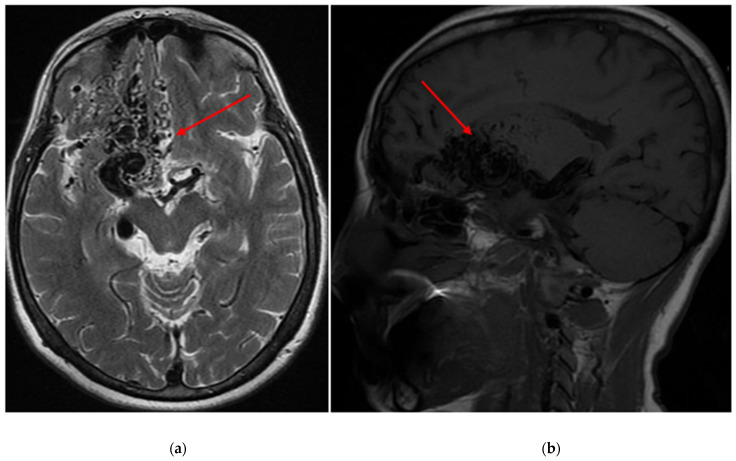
(a) An axial T2 magnetic resonance image of the brain depicts an arteriovenous malformation (AVM) situated within the right frontal lobe (red arrows), with a Spetzler-Martin grade of IV assigned to it (b) A sagittal T1 magnetic resonance image of the brain reveals the same AVM located within the right frontal lobe, also assigned a Spetzler-Martin grade of IV.
